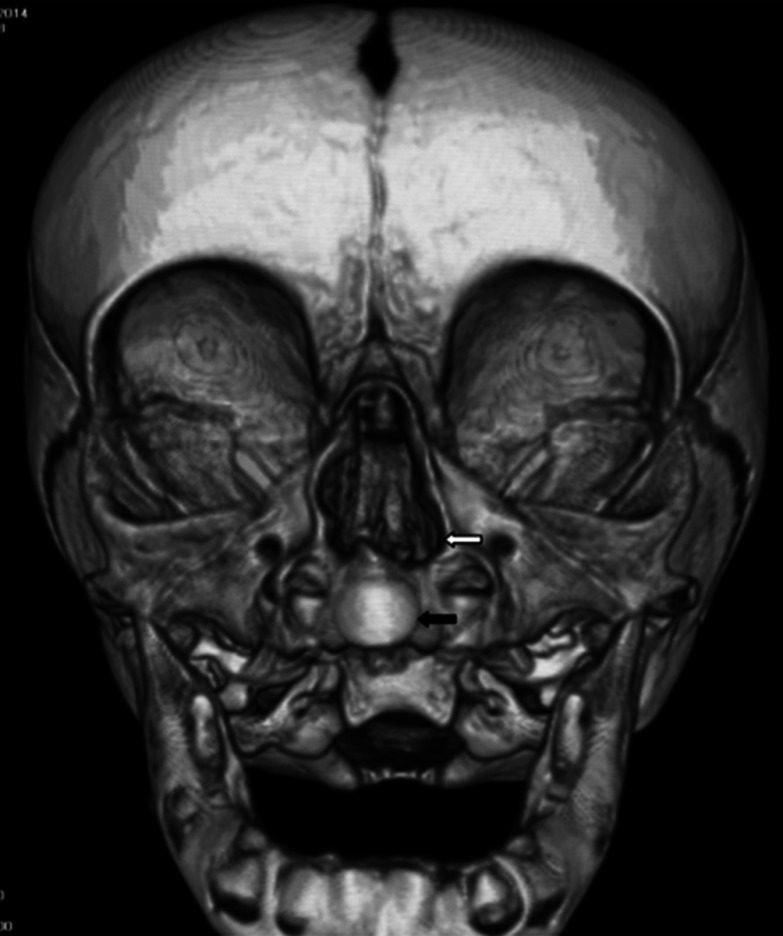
Reconstructed CT image of the widened cavity, post stenting (white arrow) with central mega-incisor (black arrow).
